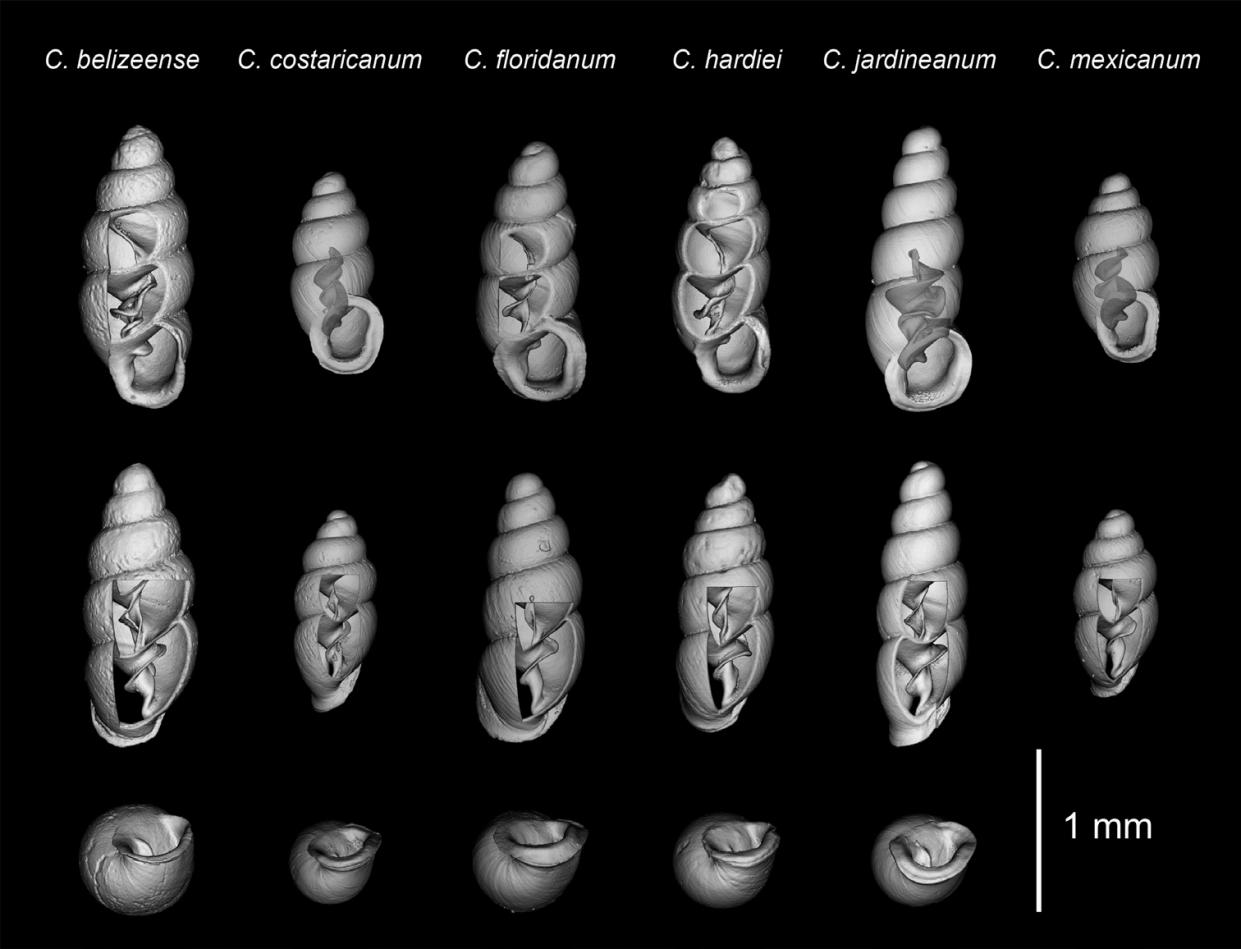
Comparative material (Jochum et al. 2017), *C.belizeense* Jochum & Weigand, 2017 paratype (NMBE 549924/8), *C.costaricanum* E. von Martens, 1898 (RBINS 10591), *C.floridanum* Clapp, 1918 (CM 46540), *C.hardiei* Jochum & Weigand, 2017 paratype (NMBE 549921/8), *C.jardineanum* (Chitty, 1853) (AJC 2321), *C.mexicanum* Pilsbry, 1891 (AJC 2092). CT images showing columellar apparatus, configuration of the columellar lamella and umbilical perspectives showing peristome configuration in allied Central American, Southeast USA and Caribbean species. Scale bar: 1 mm.
